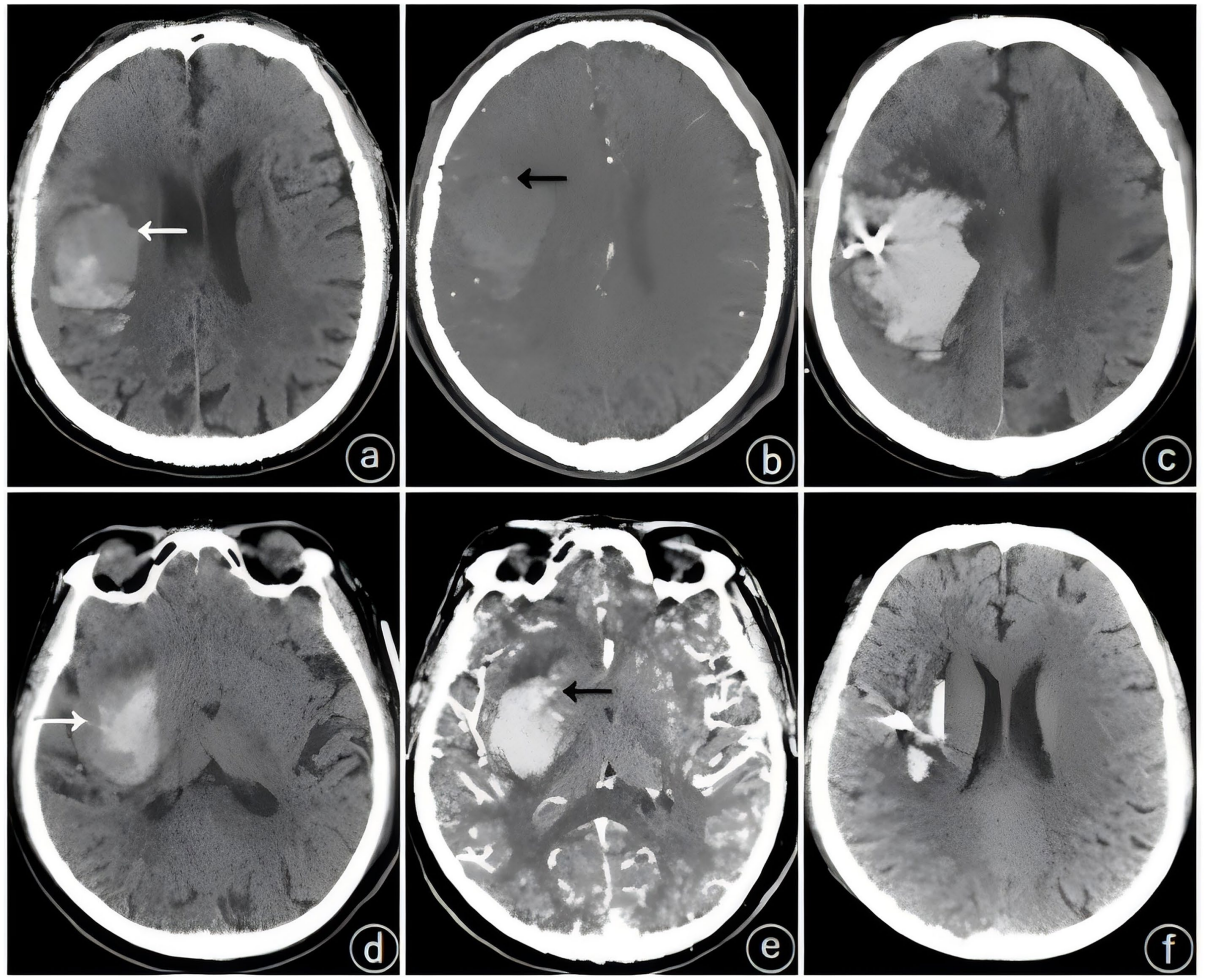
Typical cases of spot sign (black arrow) combined with blend sign (white arrow). (a–c) Patient: male, 77 years old, (a) initial hematoma of 36 mL; blend sign on computed tomography (CT); (b) spot sign on computed tomographic angiography (CTA) within 24 h; (c) HE on postoperative repeat computed tomography (CT); (d) Patient: male, 66 years old; initial hematoma of 27 mL; blend sign on computed tomography (CT); (e) spot sign on computed tomographic angiography (CTA) within 24 h; (f) no hematoma expansion (HE) on postoperative repeat computed tomography (CT).
